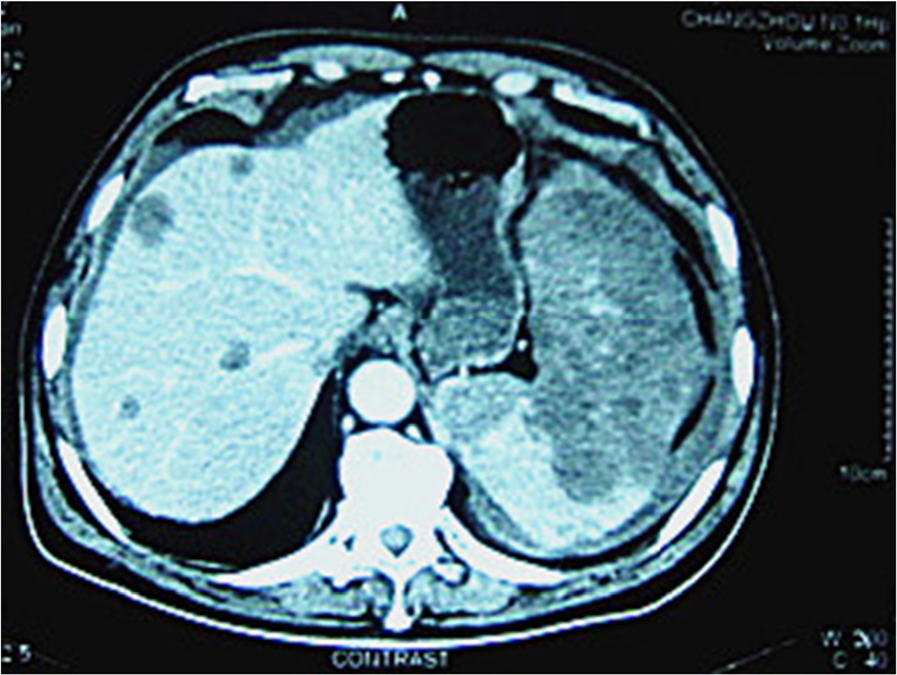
Enlarged spleen completely replaced by low-density tumor tissue and multiple hypervascular metastases in liver. Contrast-enhanced image in the portal venous phase computed tomography scan showing the enlarged spleen completely replaced by tumor tissue with low density and multiple hypervascular metastases in the liver with sizes varying from 5 mm to 2 cm. Some free fluid around the liver and spleen was also found, indicating intraperitoneal hemorrhage.
